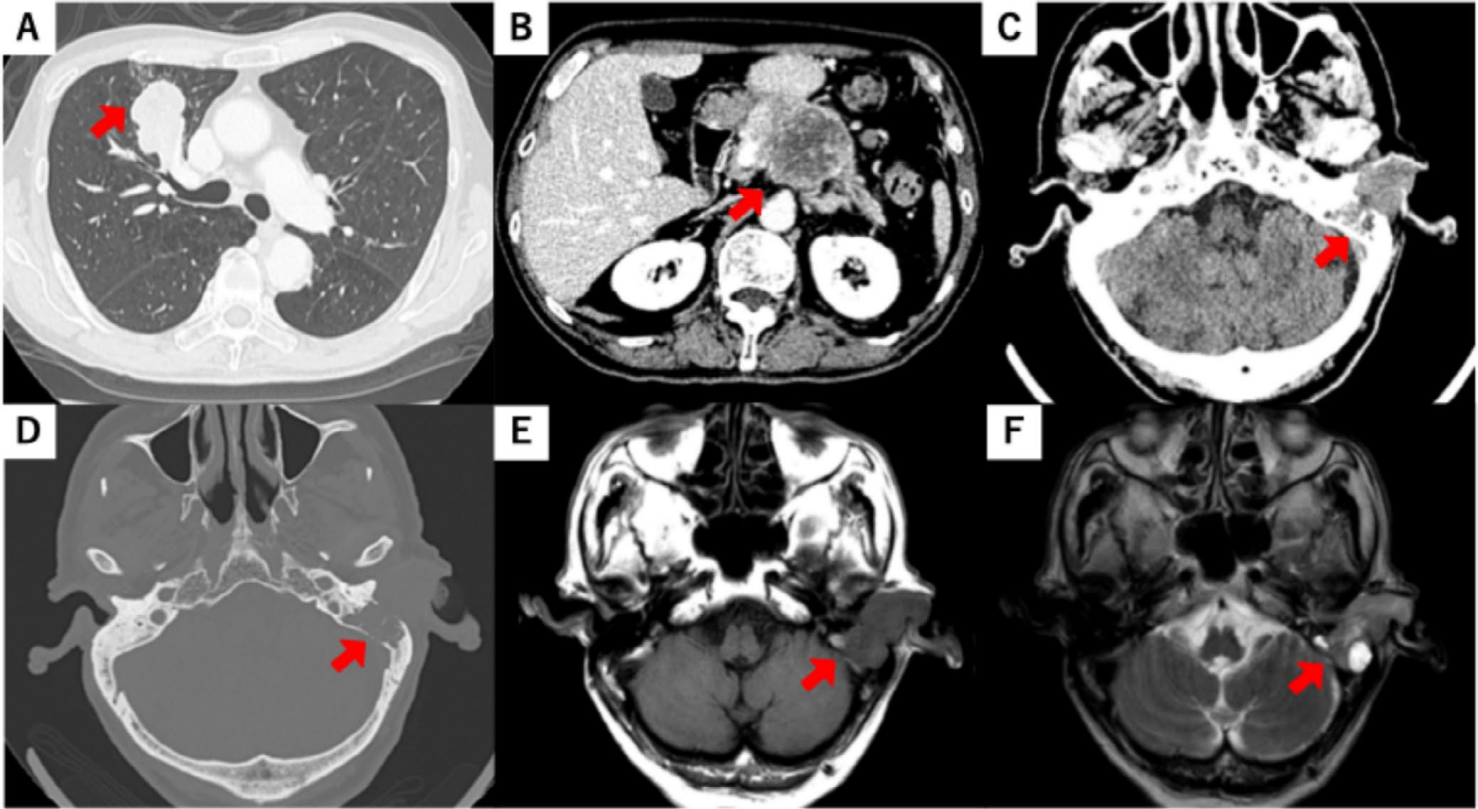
Computed tomography findings. (A) The mass in right upper lobe of lung. (B) The mass in the pancreatic body. (C) The mass in the left external auditory canal. (D) Destruction of left mastoid. Magnetic resonance imaging findings. (E) T1‐weighted imaging. (F) T2‐weighted imaging.
